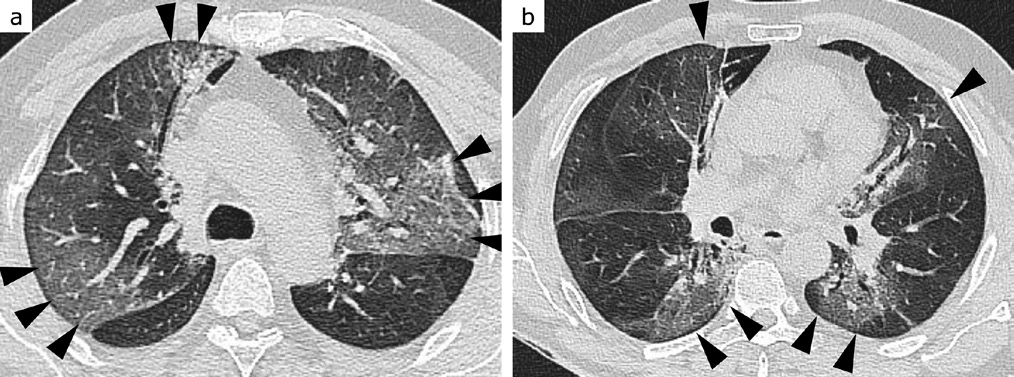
CO-RADS 5, ground-glass type. 54-year-old patient with headache, general weakness, fever and cough starting 3 days before the CT scan. Highly elevated C-reactive protein level, moderately elevated leukocyte count and lymphopenia. Geographic ground-glass opacities (arrowheads, a and b) extending from the subpleural space to central areas in both upper and lower lung zones, indicating COVID-19 pneumonia. RT-PCR for SARS-CoV-2 returned positive
